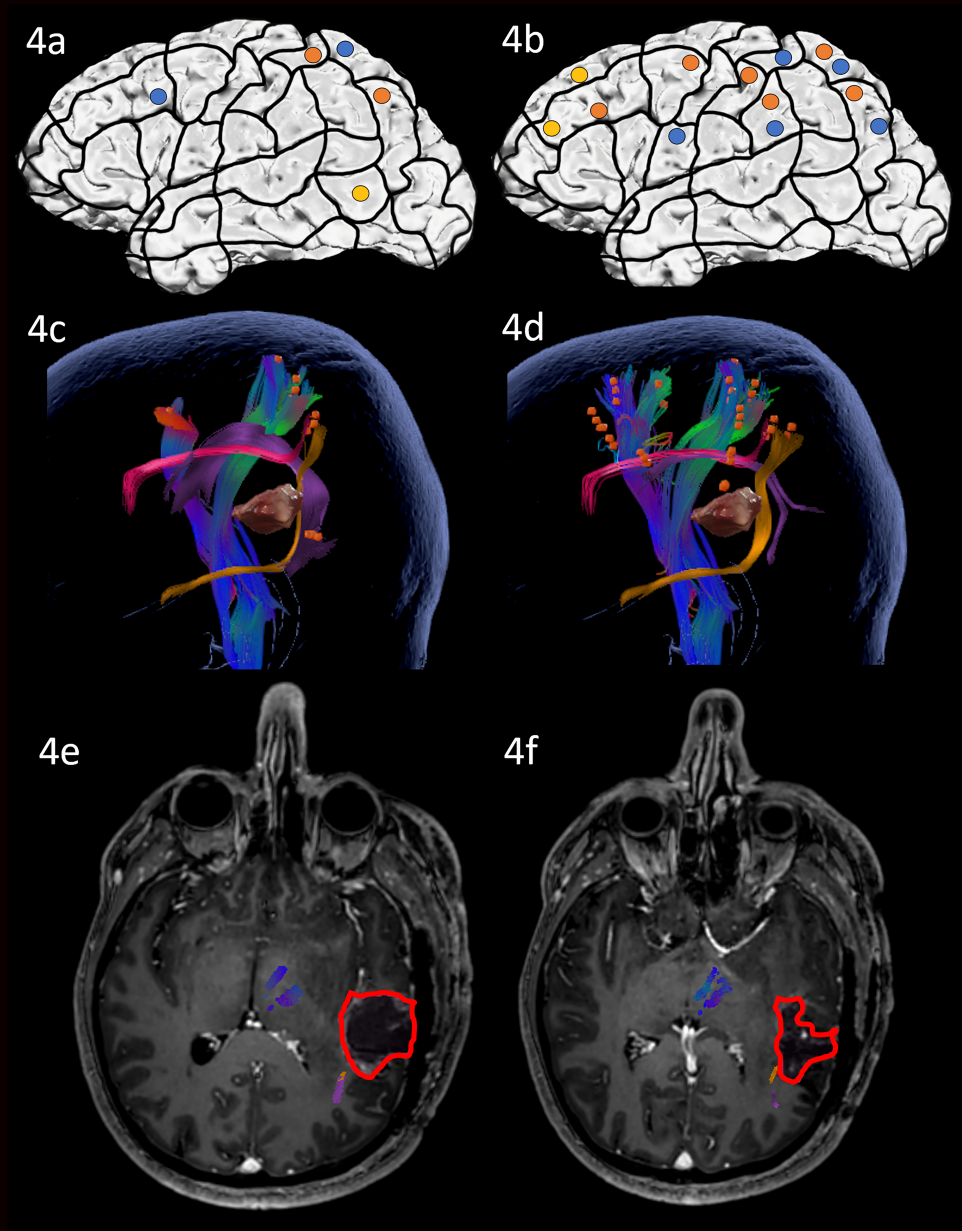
Case 4. Left panels show ON results, right panels AN results. Panels (A, B) nTMS stimulation sites that induced errors; no response and hesitations in orange, semantic errors in yellow and performance errors in blue. Panels (C, D) Fiber tracking results including the lesion, nTMS cortical endpoints in orange and color-coded tracts with CNT in direction-coded, here dark blue, AF in purple, SLF in pink, and ILF in yellow. Panels (E, F) Overlap of tracking and postoperative T1-weighted imaging after elastic fusion with the resection cavity marked in red.
